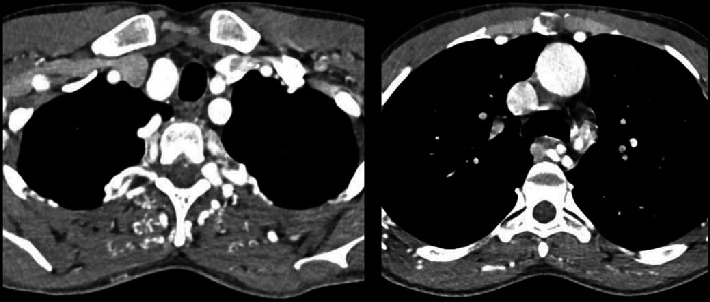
Axial postcontrast CT scan images at the level of the upper thoracic cavity, showing multiple tortuously enlarged collateral vessels in the chest wall and mediastinum.
